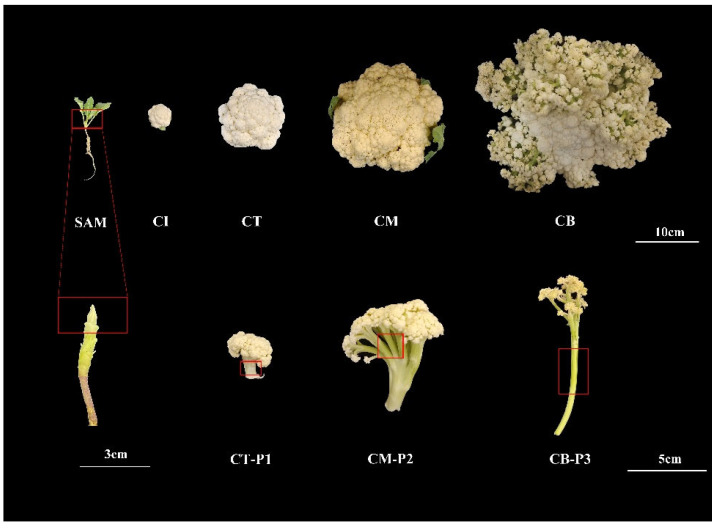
Phenotypes of cauliflower curd and peduncle development. SAM samples were taken from 25-day-old seedlings at the four-true-leaf stage. Curd samples were collected at four stages (CI: curd initiation stage; CT: curd thickening stage; CM: curd maturation stage; CB: curd bolting stage), and peduncle samples at three corresponding stages (CT-P1: peduncle at expansion stage; CM-P2: peduncle at maturation stage; CB-P3: peduncle at elongation stage).
